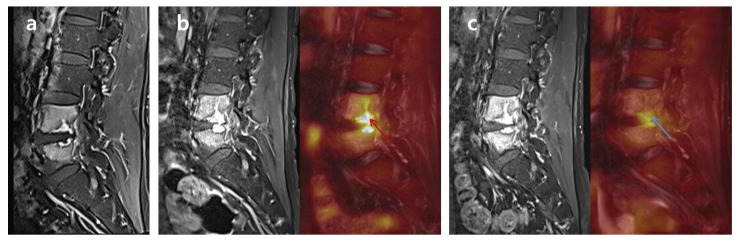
Differences in FDG uptake between ‘Non-cured’ and ‘Cured’ FDG-PET/MRIs. A 54-year-old male patient with lumbar PVO on L4-5 was treated with vancomycin and ciprobay for 46 days. The first clinical assessment on the 25th day of antibiotic therapy presented sustained back pain, intermittent fever, and a CRP/ESR of 1.399/64 (‘Non-cured’). Compared to the initial MRI (a) performed at the other hospital, the first FDG-PET/MRI (b) revealed an elevated FDG uptake (red arrow; SUV_max_ 6.58) and progression of the PVO lesion. After additional antibiotic therapy, the patient showed improved back pain and no fever, with a CRP/ESR of 0.325/31 in the second clinical assessment on the 46th day of antibiotic therapy (‘Cured’). The second FDG-PET/MRI (c) also shows markedly decreased FDG uptake and a reduced territory of the PVO lesion (blue arrow; SUV_max_ 4.3). However, determination of the therapeutic response seemed to be impossible based on the MRI due to the continuous enhancement of the PVO lesion even after successful treatment. FDG ^18^F, fluorodeoxyglucose; FDG-PET/MRI ^18^F, fluorodeoxyglucose positron emission tomography/magnetic resonance imaging; PVO, pyogenic vertebral osteomyelitis; CRP, C-reactive protein (mg/dL); ESR, erythrocyte sedimentation rate (mm/h); SUV_max_, maximum standardized uptake value of FDG.
